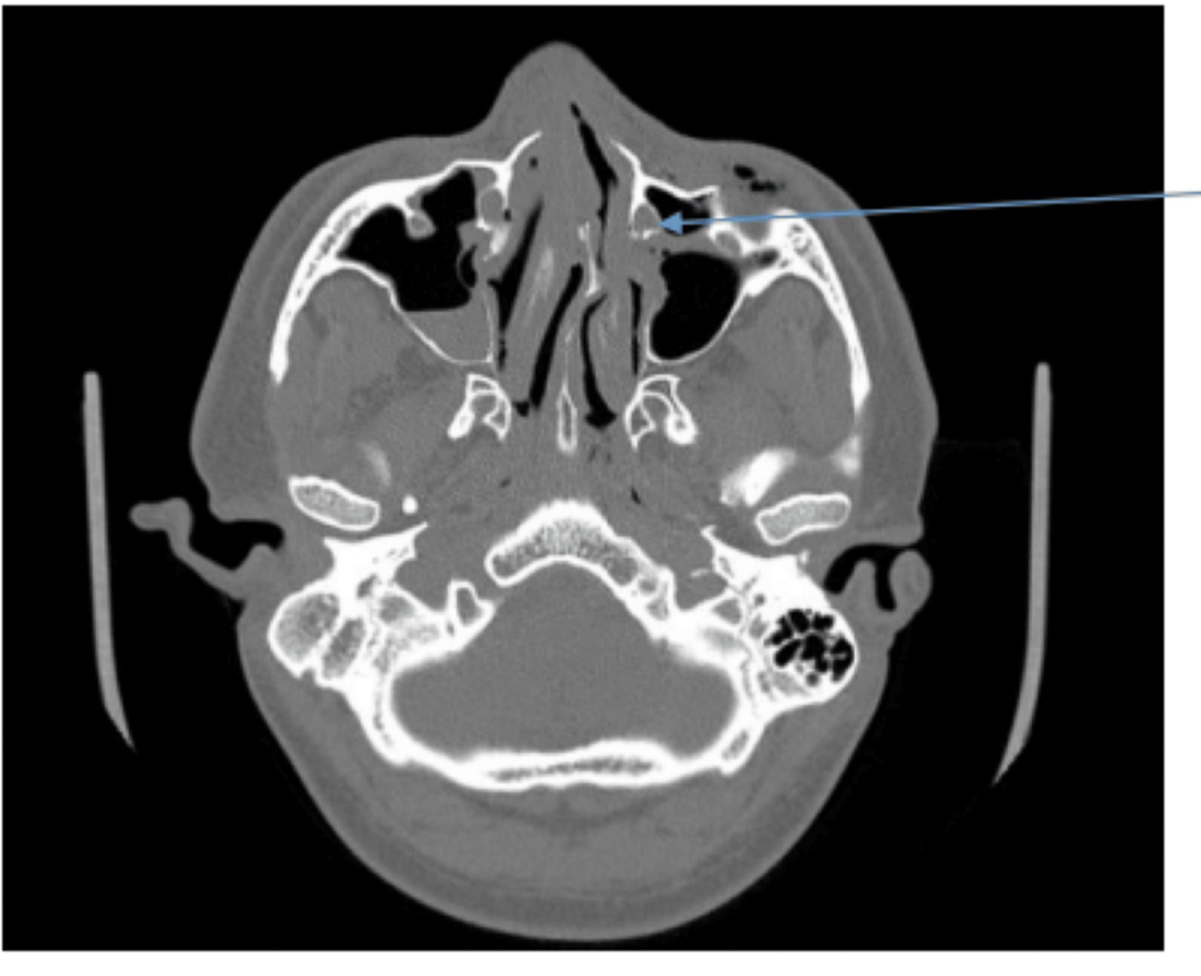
The axial section of a computed tomography scan of a fronto-naso-orbito-ethmoid fracture shows a collapse in the contour of the bony nasolacrimal canal. Arrow points out the bony nasolacrimal canal in axial view.
